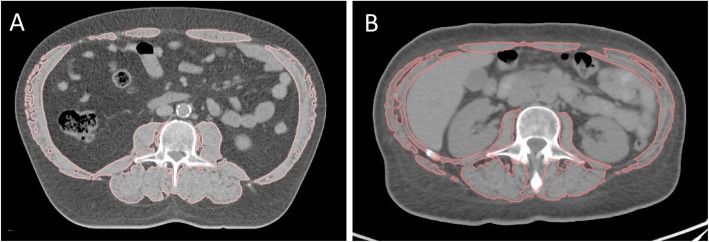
Cross-sectional abdominal CT on the level of the third lumbal vertebra. Skeletal muscle surface is delineated in a non-sarcopenic patient (a) and in a sarcopenic patient (b)
